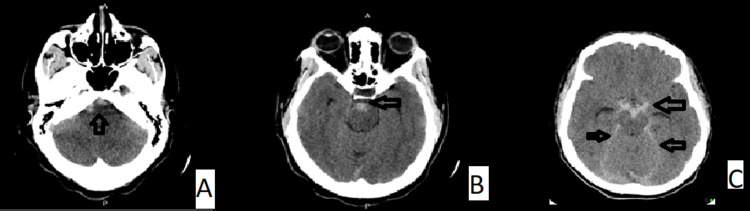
Axial slides of plain CT brain showing nonaneurysmal SAH into the prepontine, perimesencephalic, and interpeduncular cisterns.
